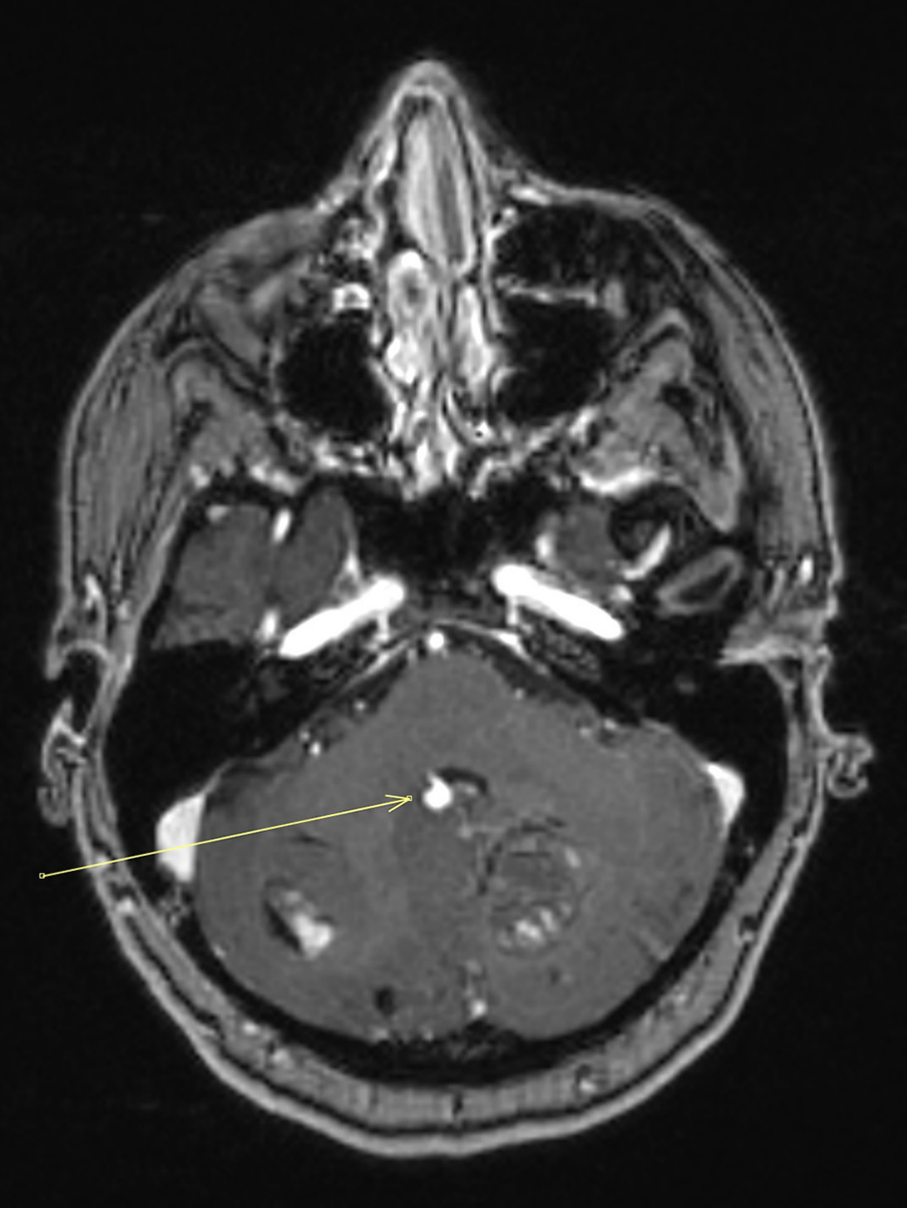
Post contrast *T*_1_ weighted stealth MRI sequence demonstrating bilateral enhancing cerebellar lesions and an avidly enhancing aneurysm arising adjacent the posterior wall of the fourth ventricle (arrow). The lesions are suspicious for metastases.
